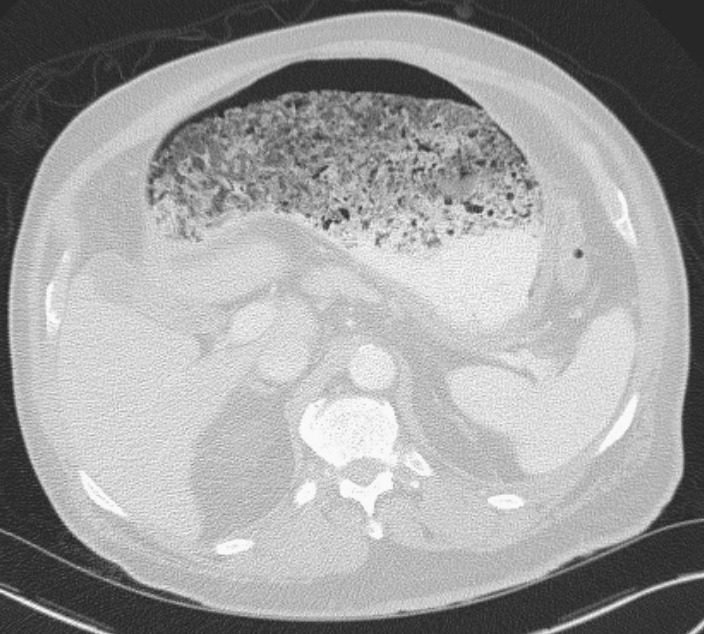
CT Abdomen showing the large amount of gastric contents consistent with a bezoar, later confirmed to be a phytobezoar.
